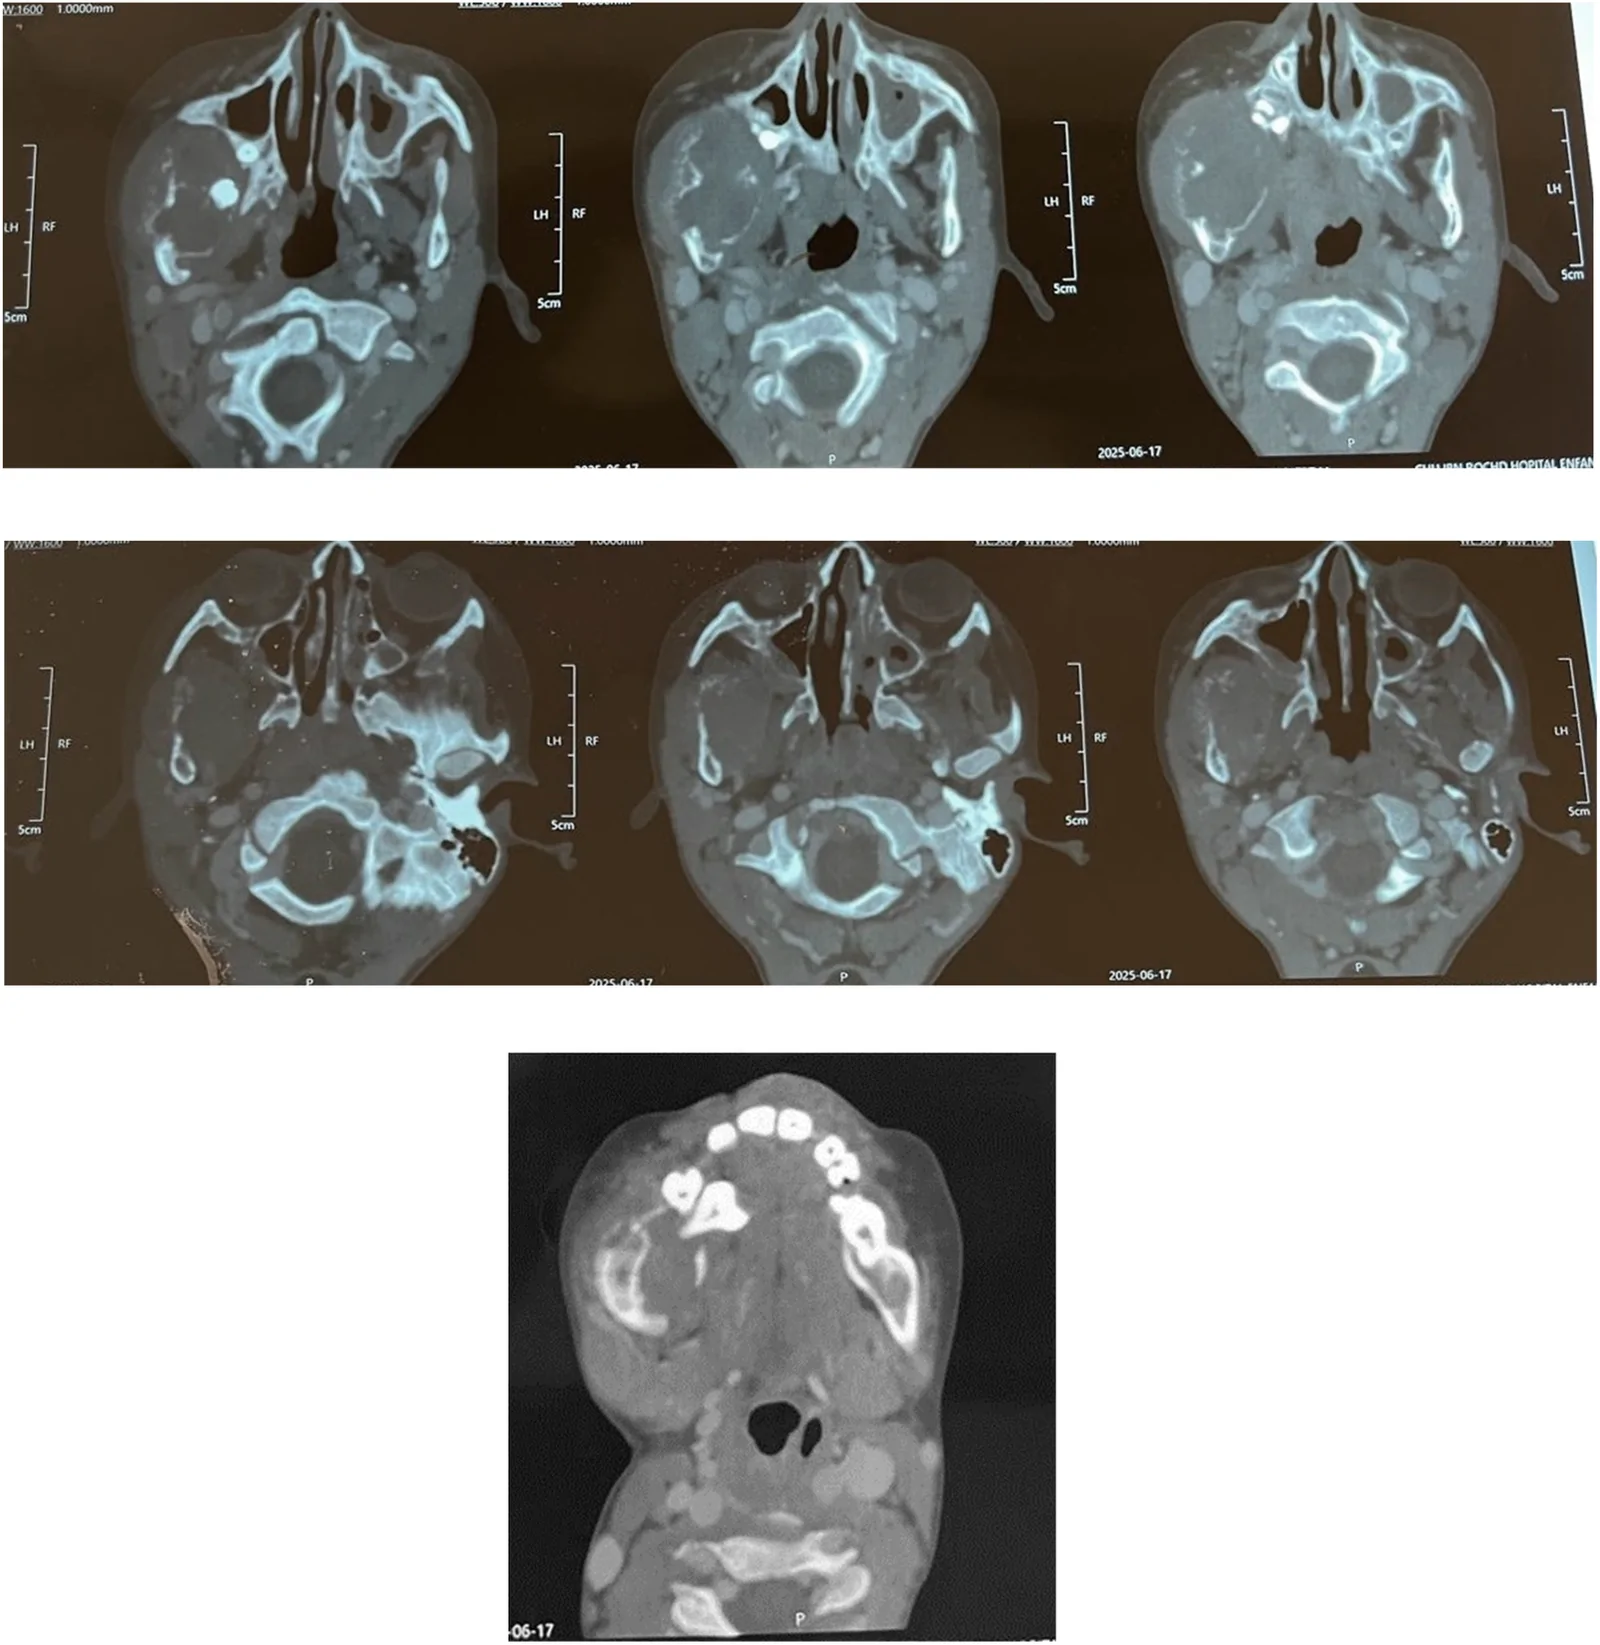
Sequential axial CT scans of the facial skeleton showing: An extensive multilocular osteolytic lesion of the right mandibular body and ramus. Marked cortical bone destruction with soft tissue extension. A lesion measuring approximately 4–5 cm in transverse diameter and 3–4 cm in anteroposterior diameter. Lingual displacement of tooth 46. Significant facial asymmetry.
